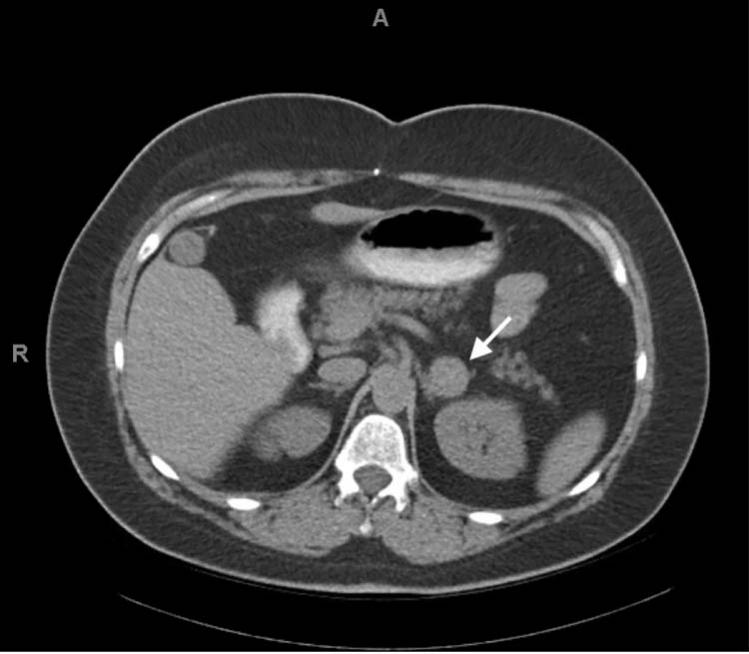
CT abdomen and pelvis without contrast demonstrating high density (40 HU), 2 cm left adrenal incidentaloma.
